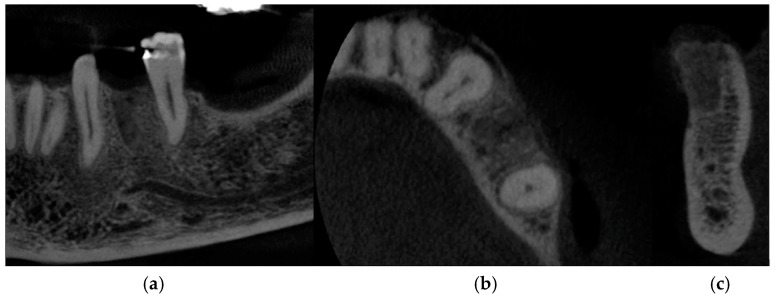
CBCT images at 4 months after the surgery: (a) frontal section, (b) transversal section, and (c) sagittal section.
